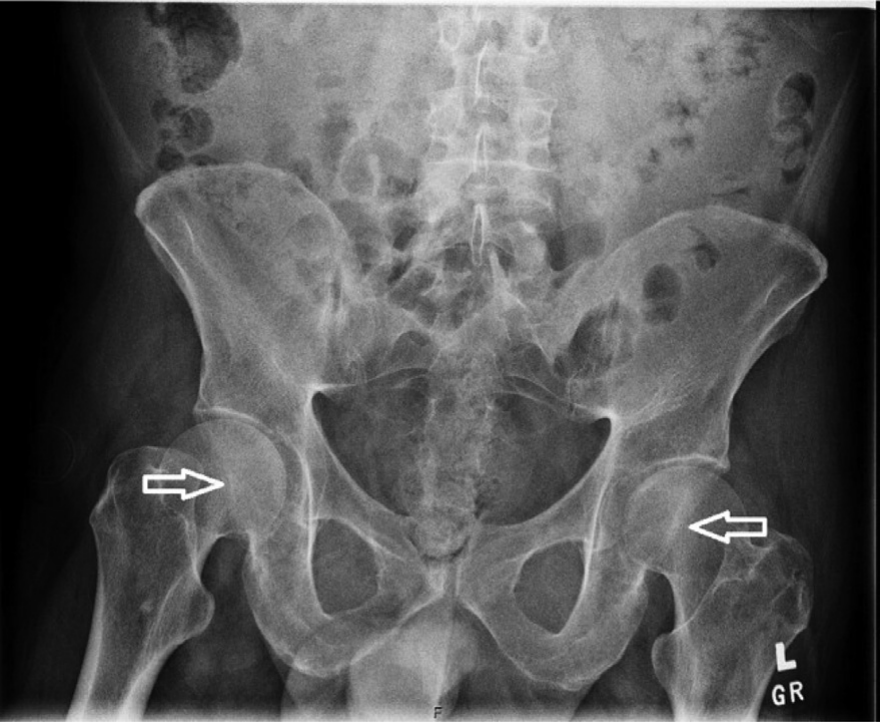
Key image from bilateral hip and pelvis X-rays with mild sclerosis of the femoral heads originally concerning for early-stage avascular necrosis (nonshaded arrows).
